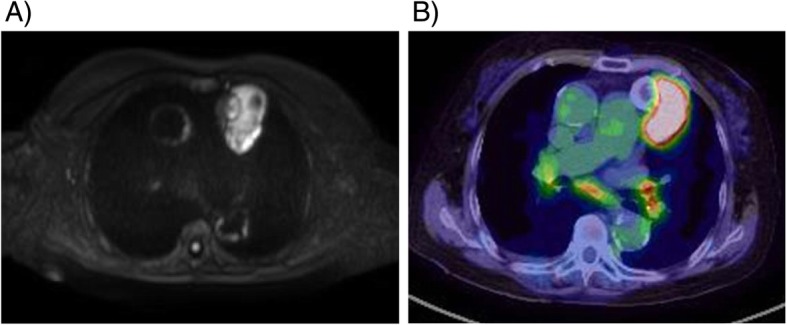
a Diffusion-weighted image acquired by magnetic resonance imaging. The suppression of diffusion was strong only outside the tumor. b 2-Deoxy-2-fluoro-D-glucose positron emission tomography of the tumor. FDG uptake was evident only outside the tumor. Tiny mediastinal lymph nodes also showed 18FDG uptake
